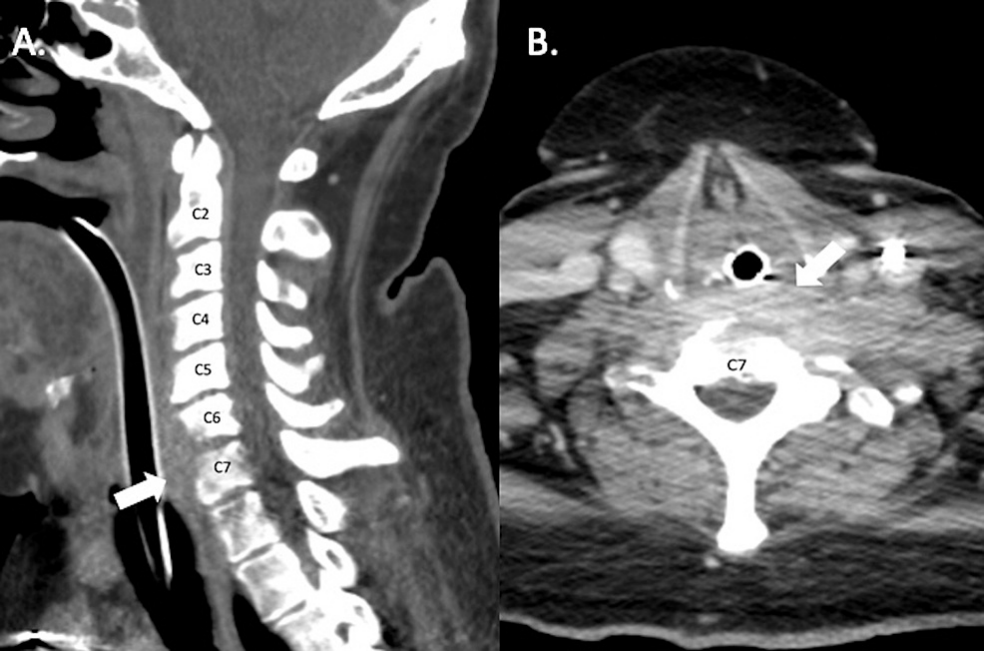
Initial Computed Tomography Scan of the Cervical Spine A. Sagittal view of computed tomography scan with intravenous contrast performed before obtaining computed tomography myelogram that demonstrates the erosion of the C6 and C7 vertebral bodies anteriorly with prevertebral phlegmon and a 7 mm prevertebral fluid collection concerning for abscess (white arrow). B. Axial view of C6-C7 level displaying the prevertebral collection (white arrow).
